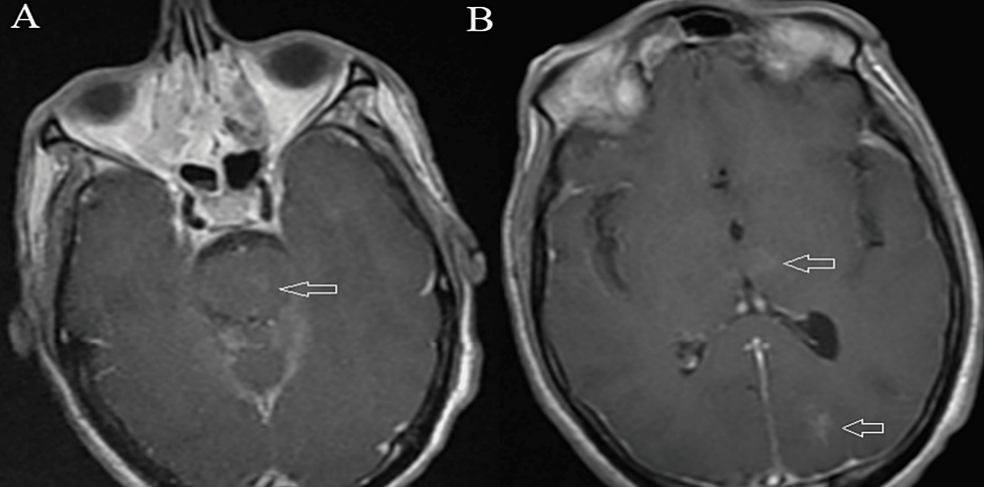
Cranial magnetic resonance imaging on contrast-enhanced T1-weighted images showing small areas of enhancement in the pons (A) (arrow), thalami, and left occipital region (B) (arrows).
